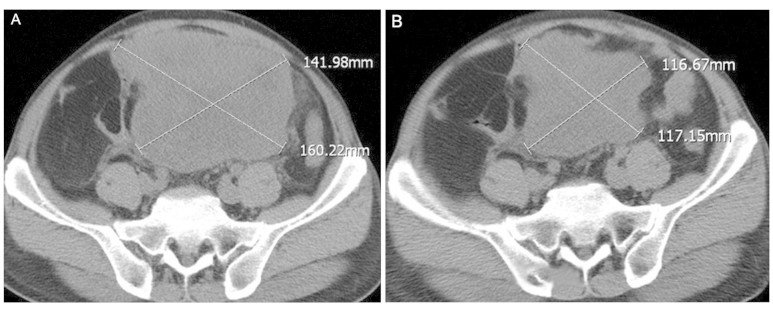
(A) Re-evaluated computed tomography (CT) scan revealing a notable enlargement of the globular, non-adipose tissue eight days subsequent to the inital scan. The tumor measured 14.2×16.0 cm. (B) The initial CT scan revealed that the tumor measured 11.6×11.7 cm.
